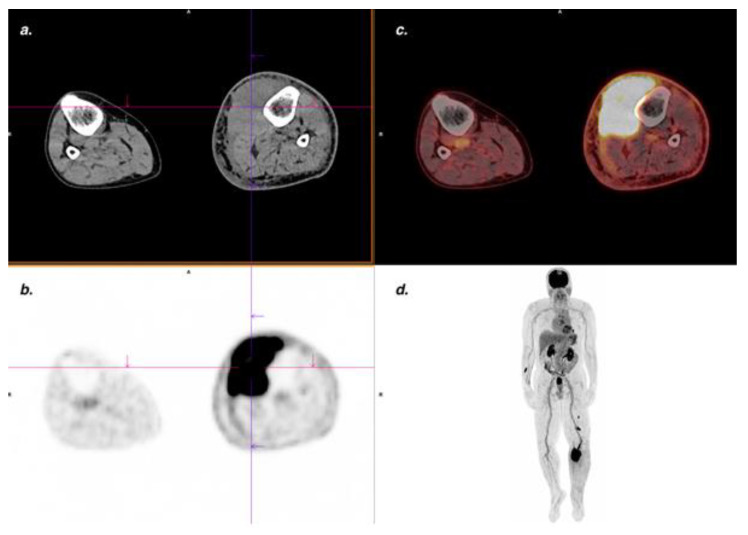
Endemic KS. Suspected clinical recurrence with increased lymphedema of left lower limb. The FDG PET/CT showed a voluminous, intensely hypermetabolic (SUV_max_ 26.7) intramuscular lesion confirmed by MRI and pathological analysis. (a) CT transaxial slice; (b) PET transaxial slice; (c) Fused PET/CT transaxial slice; (d) Maximum intensity projection (MIP) PET images.
